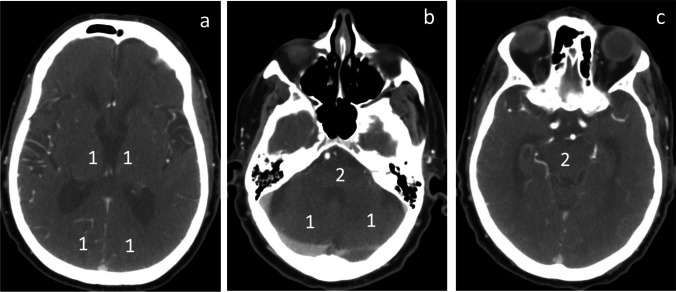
Posterior circulation Acute Stroke Prognosis Early CT Score (pc-ASPECTS) on CTA source image (CTASI). From 10 points, 1 point each (as indicated) is subtracted for hypoattenuation in the left or right thalamus, posterior cerebral artery territory (a), or cerebellar hemisphere (b), respectively, and 2 points each for hypoattenuation in any part of pons (b) or midbrain (c). pc-ASPECTS = 10 indicates a normal scan
